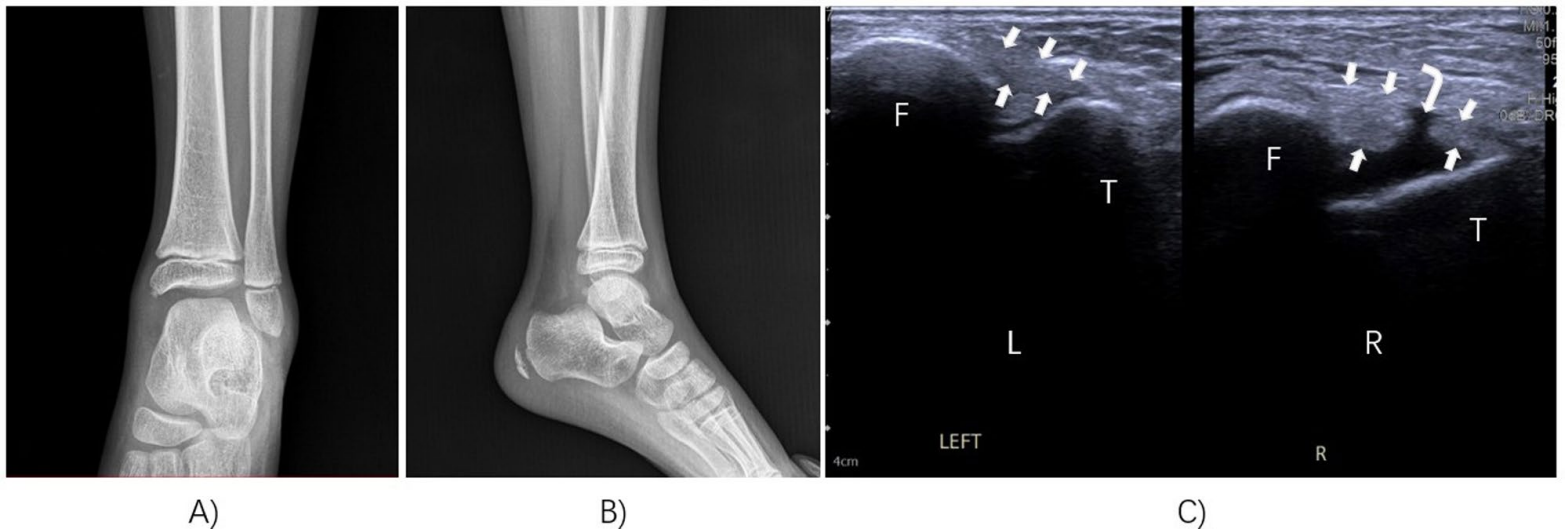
Anterior talofibular ligament injury was positive on ultrasonography. (A) Anteroposterior X-ray radiographs of the ankle. (B) Lateral X-ray radiographs of the ankle. (C) Ultrasound examination of the ankle was performed and compared with the contralateral side. *R*, right; *L*, left; *F*, fibula; *T*, talus; arrowhead, anterior talofibular ligament; right angle arrow, the site of injury to the anterior talofibular ligament
